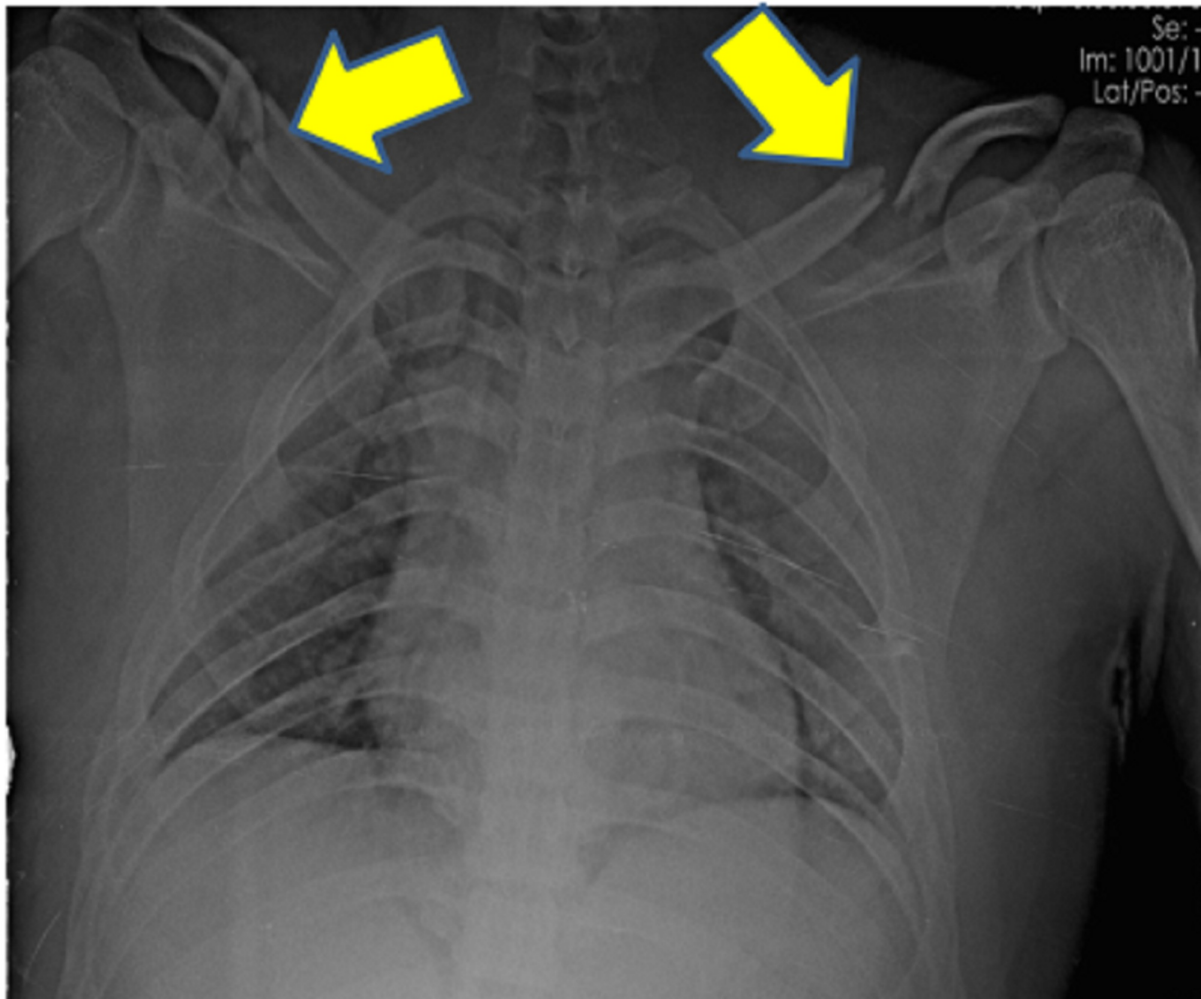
Plain radiographs of the chest with bilateral shoulder anteroposterior view showing bilateral clavicle fracture
